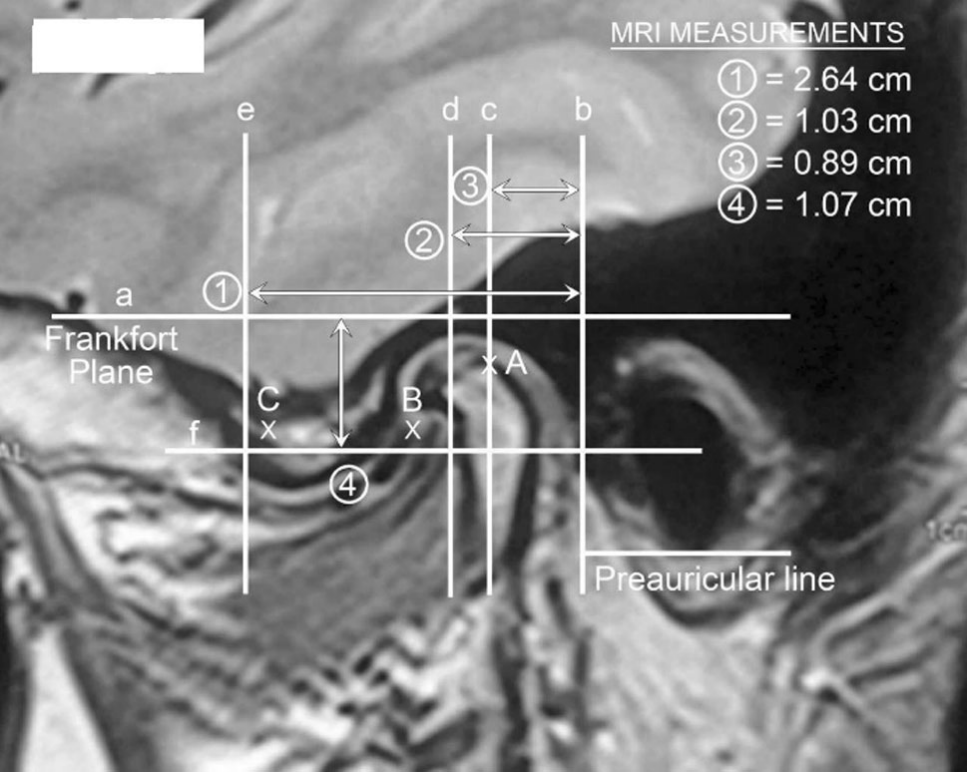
Sagittal MRI showing marking and measurements
